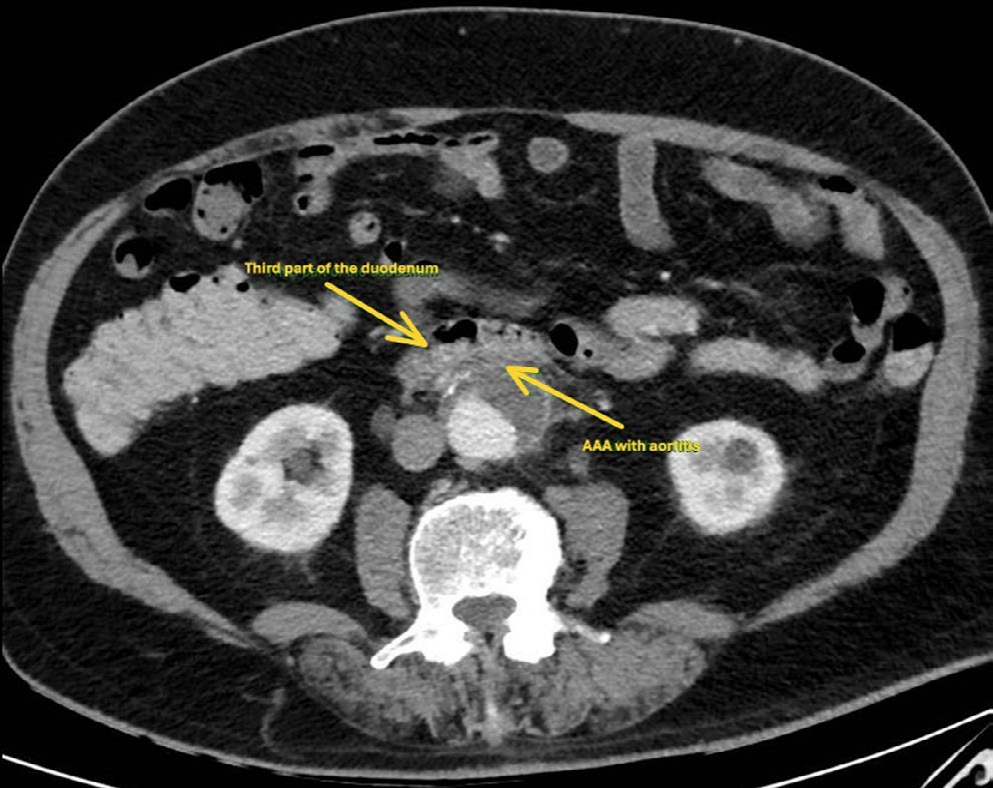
A computed tomography image of an infra‐renal saccular abdominal aortic aneurysm with features of aortitis in the aortic wall opposing the duodenum.
